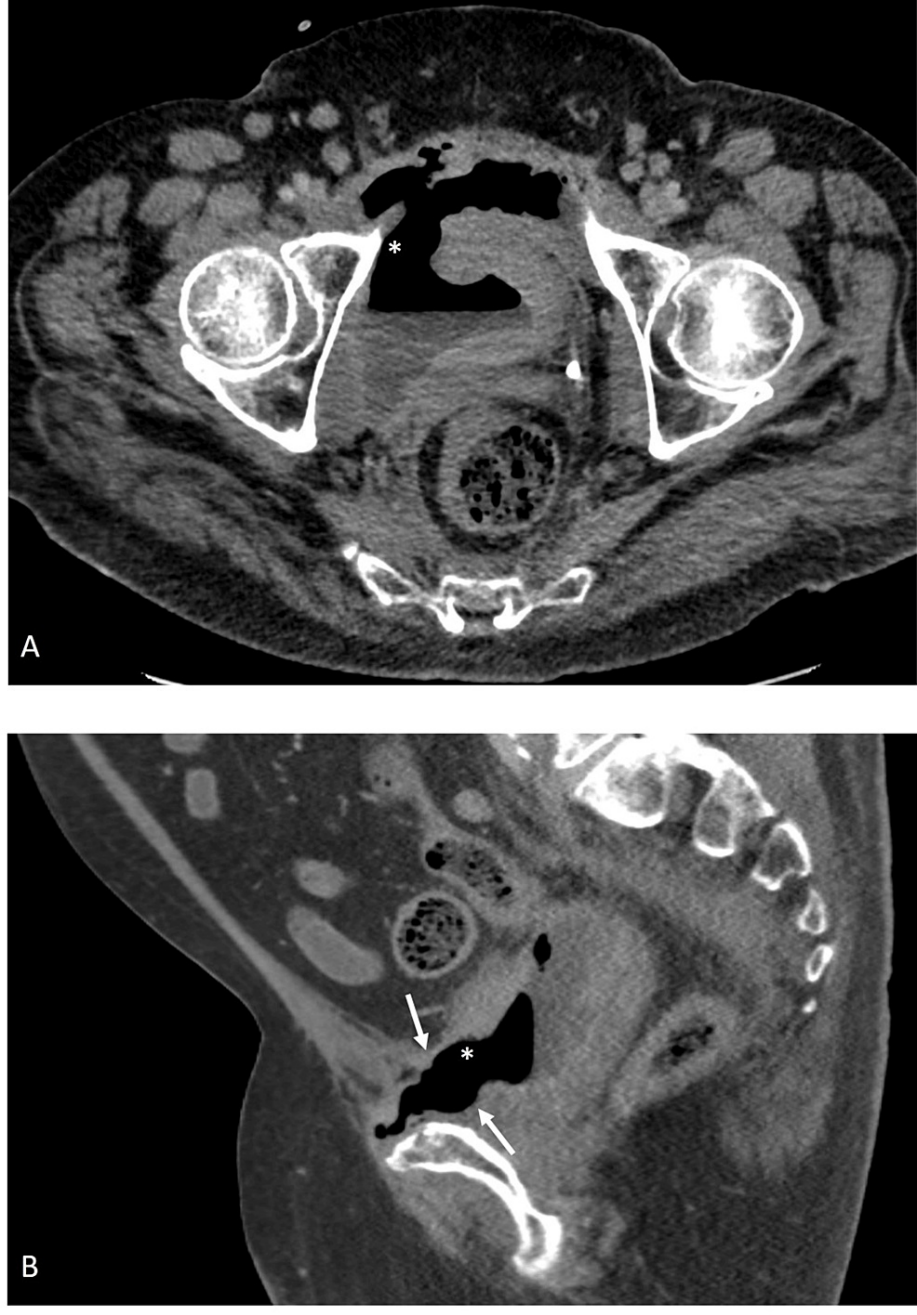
Contrast-enhanced CT images through the pelvis in axial (A) and sagittal (B) planes Contrast-enhanced CT images of the pelvis demonstrate direct fistulous communication (asterisk) between the urinary bladder lumen and the pubic symphysis (white arrows).
